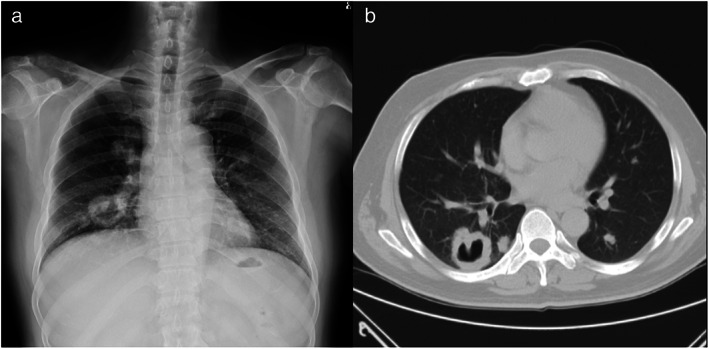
(a) Chest radiograph revealed a well‐defined mass, with a cavity over the right lower lung field. Multiple nodules were noted in the other lung fields. (b) Computed tomography revealed a well‐defined, thick‐walled cavitary mass with an irregular inner border. Two other nodules were identified
